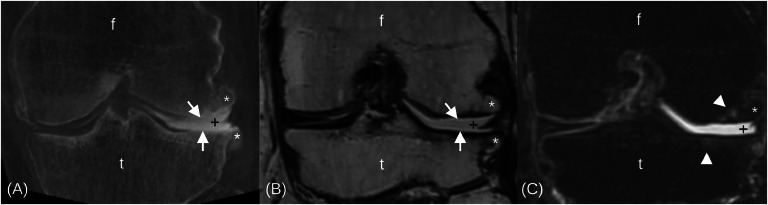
Lateral tibiofemoral osteoarthritis in a 60-year-old man in coronal orientation. CBCTA (A) depicts the full-thickness cartilage lesions of posterolateral femoral and tibial cartilage surfaces (arrows) with large tibiofemoral osteophytes (asterisks) as well as the absent, macerated, posterolateral meniscus (plus signs). PD- (B) and T2-weighted fat-saturated (C) MRI sequences confirm the CBCTA findings and show small cartilage lesion-associated subchondral bone marrow lesions (arrowheads).
